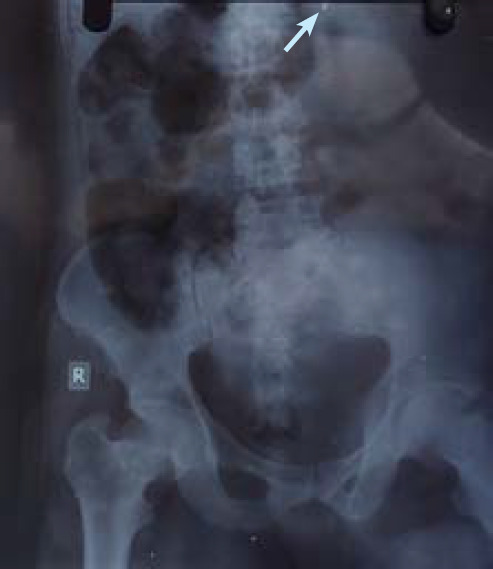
Abdominal X-ray with an anterior-posterior view revealing a hyper-dense area on the upper left side, resembling the shape of the nose of the bullet.
